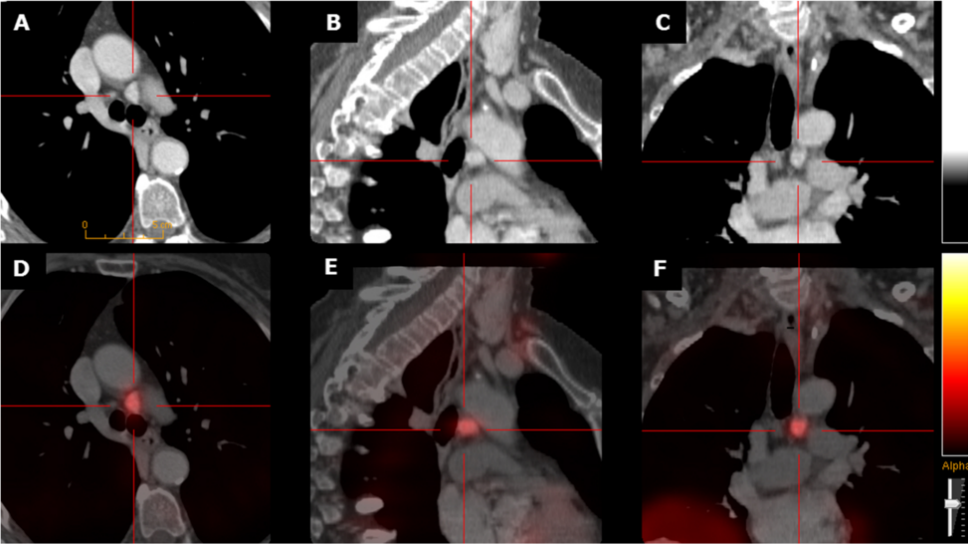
Aortopulmonary window parathyroid adenoma in patient #2: contrast-enhanced CT (CE-CT; panels A-C) and CE-CT co-registered with ^99m^Tc-MIBI SPECT (panels D-F) in axial (A, D), sagittal (B, E), and coronal (C, F) orientation.
